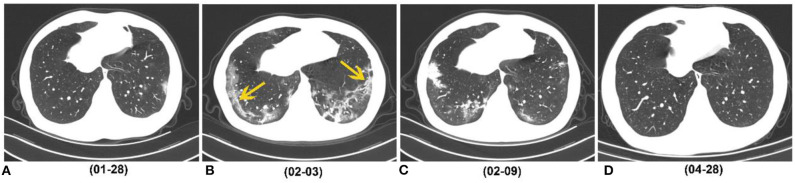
A 29-year-old man who caught a fever on January 23, 2020, received three follow-up scans after the initial chest CT. (A) Initial CT performed on January 23 (the day of symptoms onset) showed patchy GGO in the left lower lobe. (B) Chest CT performed on February 3 showed consolidation, GGO and strip-like lesions (easily misdiagnosed as fibrosis) in the bilateral lower lobes (yellow arrow). (C) Chest CT performed on February 9 showed strip-like lesions disappeared, and multiple GGO and consolidation presented in the bilateral lower lobes. (D) On April 28 (116 days after symptoms onset), CT image showed that all lesions were absorbed. COVID-19, coronavirus disease 2019; GGO, ground-glass opacity.
